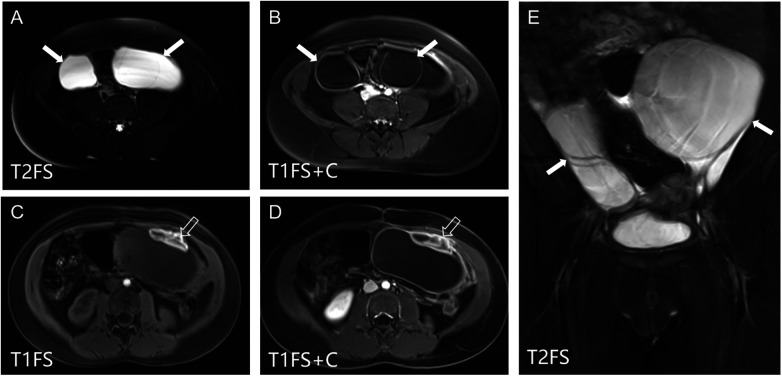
Pelvic MRI showing bilateral ovarian cysts. (A,B,E) Coronal and axial T1WI and T2WI reveal large multilocular cystic lesions in both adnexal regions (white arrows) with thin internal septations and well-defined borders. (C,D) Hemorrhagic foci within the left adnexal cyst are demonstrated by peripheral high signal intensity on T1-weighted images (hollow white arrow).
